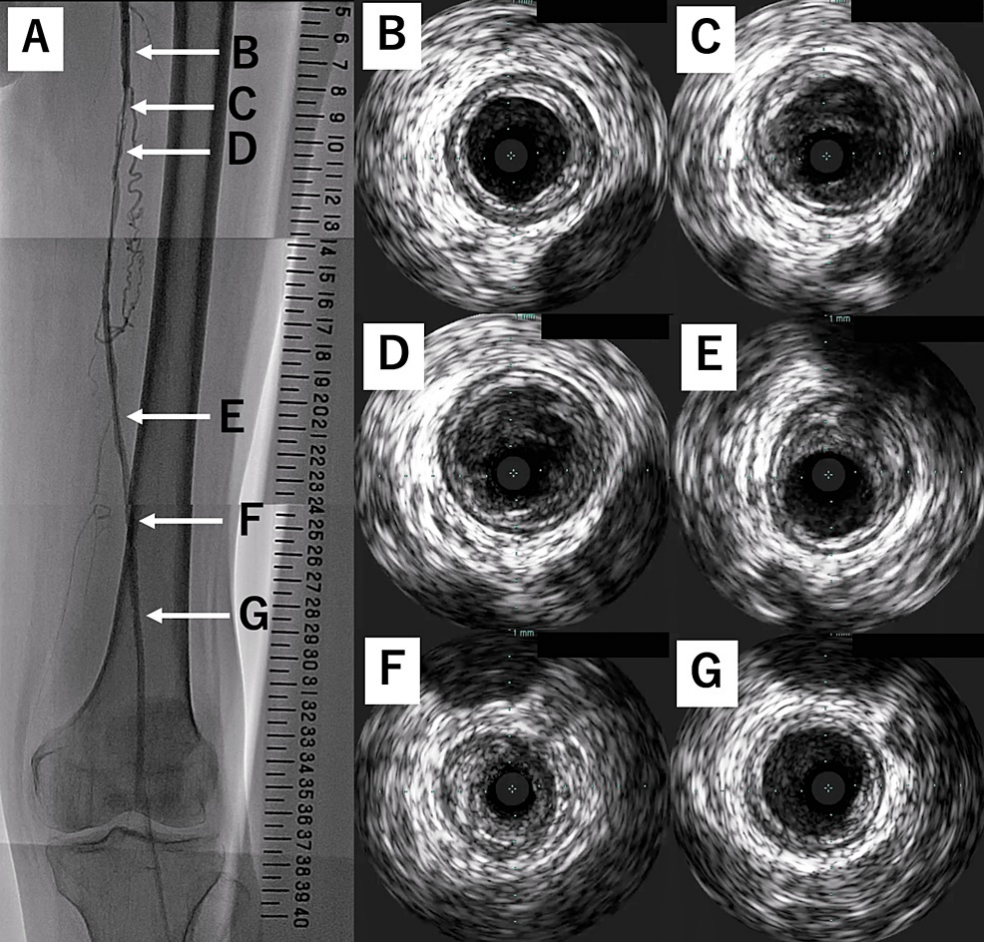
Catheter angiography and intravascular ultrasound images of the left lower limb obtained after pre-dilation. (A) Catheter angiography of the left lower limb. This image was created by merging several images into one and shows type D dissection in the middle part of the superficial femoral artery. (B-G) Intravascular ultrasound cross-sectional images from the proximal part of the superficial femoral artery to the middle part of the popliteal artery. (B) The vessel diameter is approximately 6.0 mm in the proximal reference site. (C-D) These segments are filled with thrombotic materials. (E) Mild stenosis with fibrous plaque. (F) Severe stenosis with extensive fibrous plaque. (G) The vessel diameter is approximately 6.0 mm at the distal reference site.
